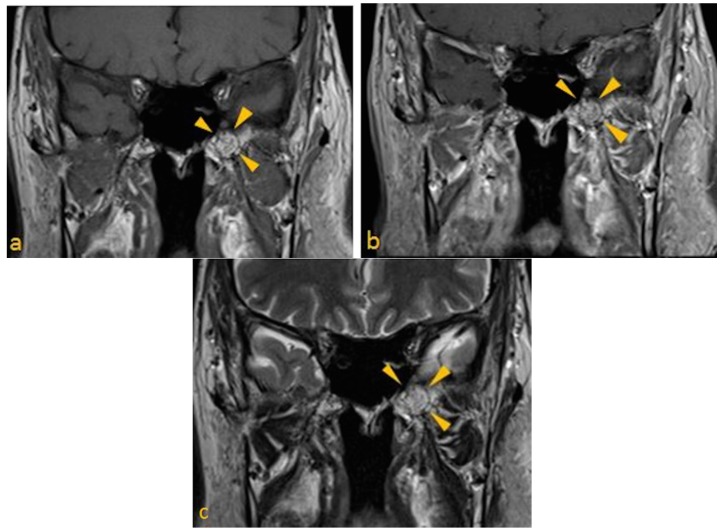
Brain MRI Paracoronal images (a) T1 pre-contrast; (b) T1 post gadolinium; and (c) T2 post gadolinium images, show arrested pneumatization of left sphenoid sinus. Note mild architectural distortion without a focal mass lesion in the marrow of the left pterygoid.
